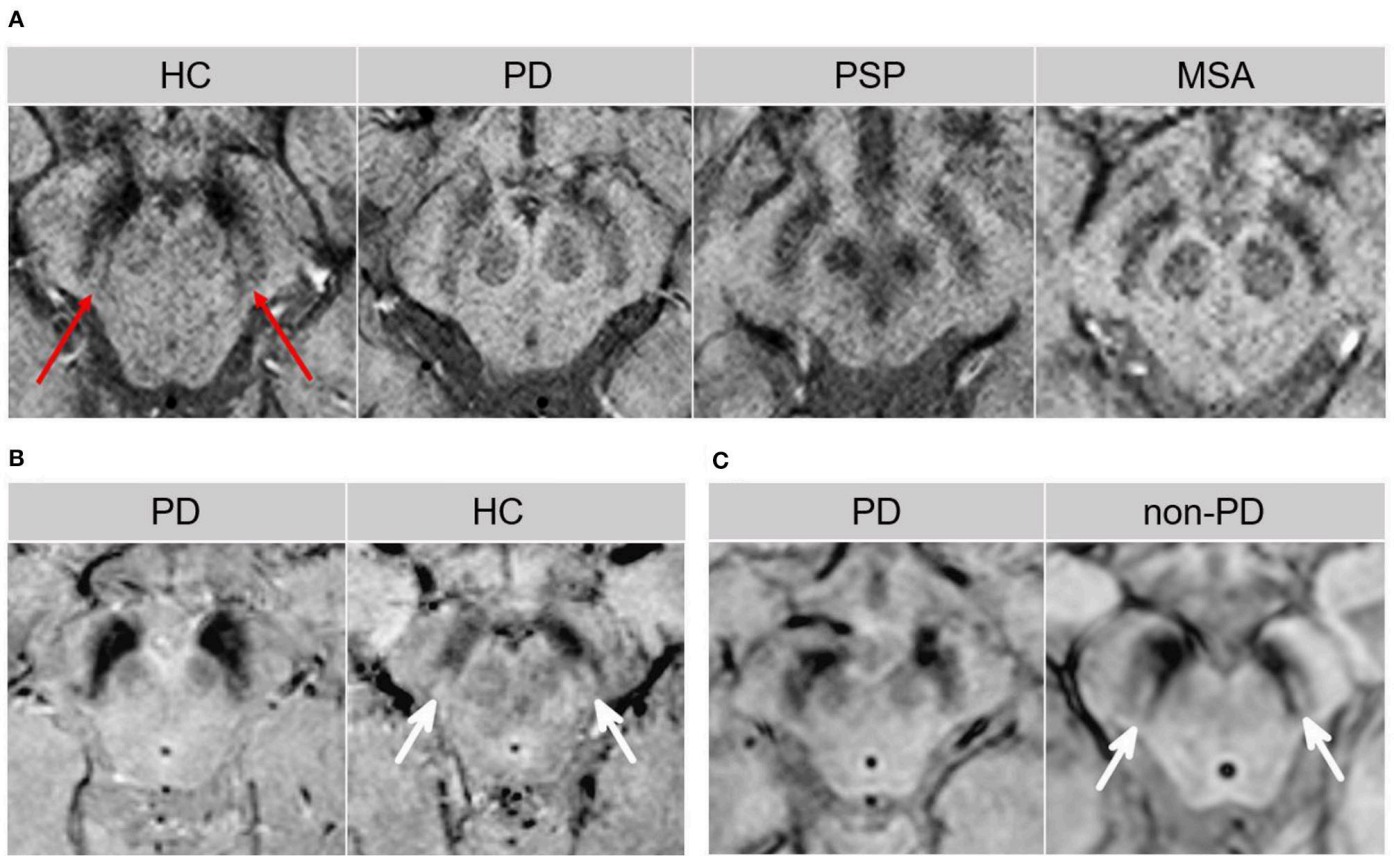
The “swallow tail” sign. All MRI presented above are taken at the level of substantia nigra in the midbrain. (A) Susceptibility-weighted MRI depicting dorsolateral nigral hyperintensity (the “swallow tail” sign, red arrows) in a healthy control. Loss of dorsolateral nigral hyperintensity can be seen in PD and may even be seen in some PSP and MSA cases on susceptibility-weighted MRI. (B) High resolution susceptibility-weighted MRI (gradient echo-echo planar imaging sequence, magnitude image) is shown for a PD patient and a control. (C) High resolution T2*/susceptibility-weighted MRI (multi-shot fast field echo-echo planar imaging sequence) is shown for a PD patient and a non-PD case who was diagnosed with aneurysmal subarachnoid hemorrhage. In both (B,C), loss of dorsolateral nigral hyperintensity (white arrows) corresponding to nigrosome-1 can be seen in PD as compared to control and a non-PD subject. (A) was adapted from Chougar et al. (40), and (B,C) were adapted from Schwarz et al. (39), under the Creative Commons Attribution License (https://creativecommons.org/licenses/by/4.0/). HC, healthy control; MSA, multiple system atrophy; PD, Parkinson's disease; PSP, progressive supranuclear palsy.
